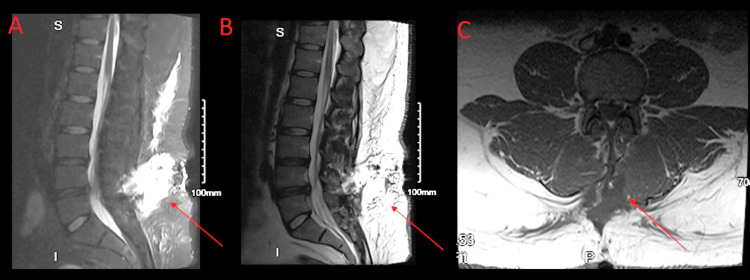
Postsurgical changes at L5-S1 with left hemilaminectomy A and B show a sagittal lumbar MRI, and C shows a coronal lumbar MRI. The arrows indicate fluid collection in the retrospinal soft tissues resulting in moderate narrowing of the thecal sac on the left, displacing the nerve roots toward the right within the canal. MRI: magnetic resonance imaging
